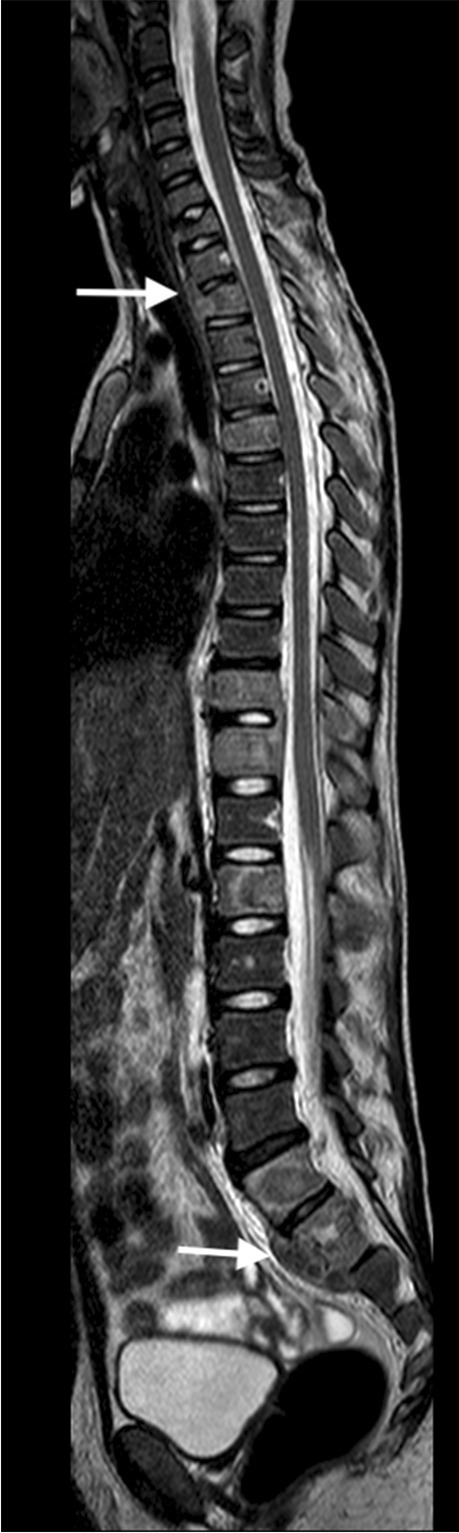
A sagittal T2 magnetic resonance image of the spine in a 7-year-old girl with tuberculous spondylitis. There is multilevel non-contiguous disease as evidenced by the increased signal in the vertebral bodies; note the anterior subligamentous spread at T1/T2 and S1/S2 (*arrows*). There is preservation of the disc height at all levels and disc signal at almost all levels
